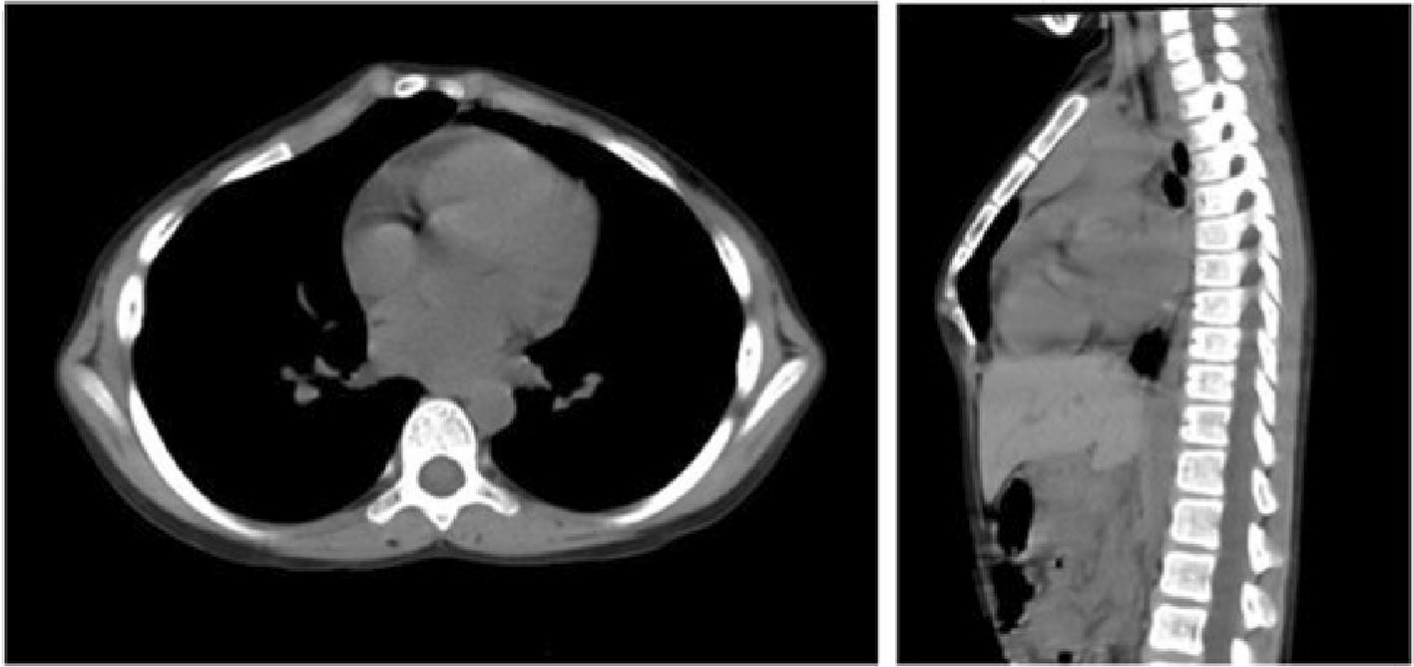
Preoperative CT scan of the chest of the paediatric patient, showing the sternum protruding forward that forms an angular deformity.
